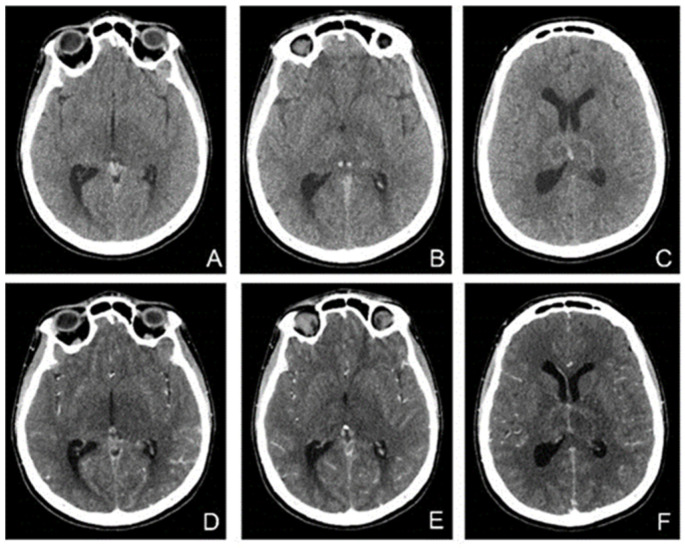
CT without contrast (A–C) and with (D–F) contrast enhancement shows hyperdensities in the Galen vein and internal cerebral veins with nonhomogeneous thalamic nuclei. After contrast enhancement, there was a slight enhancement in the veins and hypoperfusion of the thalamic nuclei.
